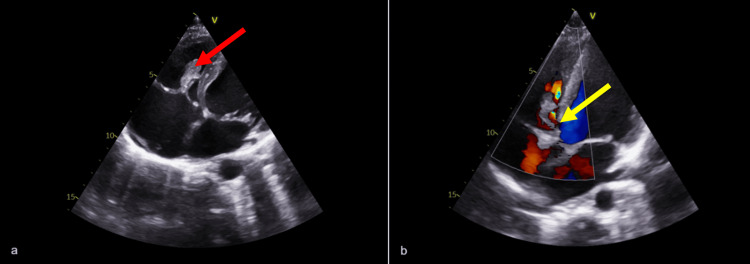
Four-chamber transthoracic echocardiogram (a) Large vegetation adherent to the tricuspid septal leaflet (red arrow) measuring 23 x 9 mm; (b) Left-to-right shunt through a small perimembranous ventricular septal defect (VSD) (yellow arrow).
